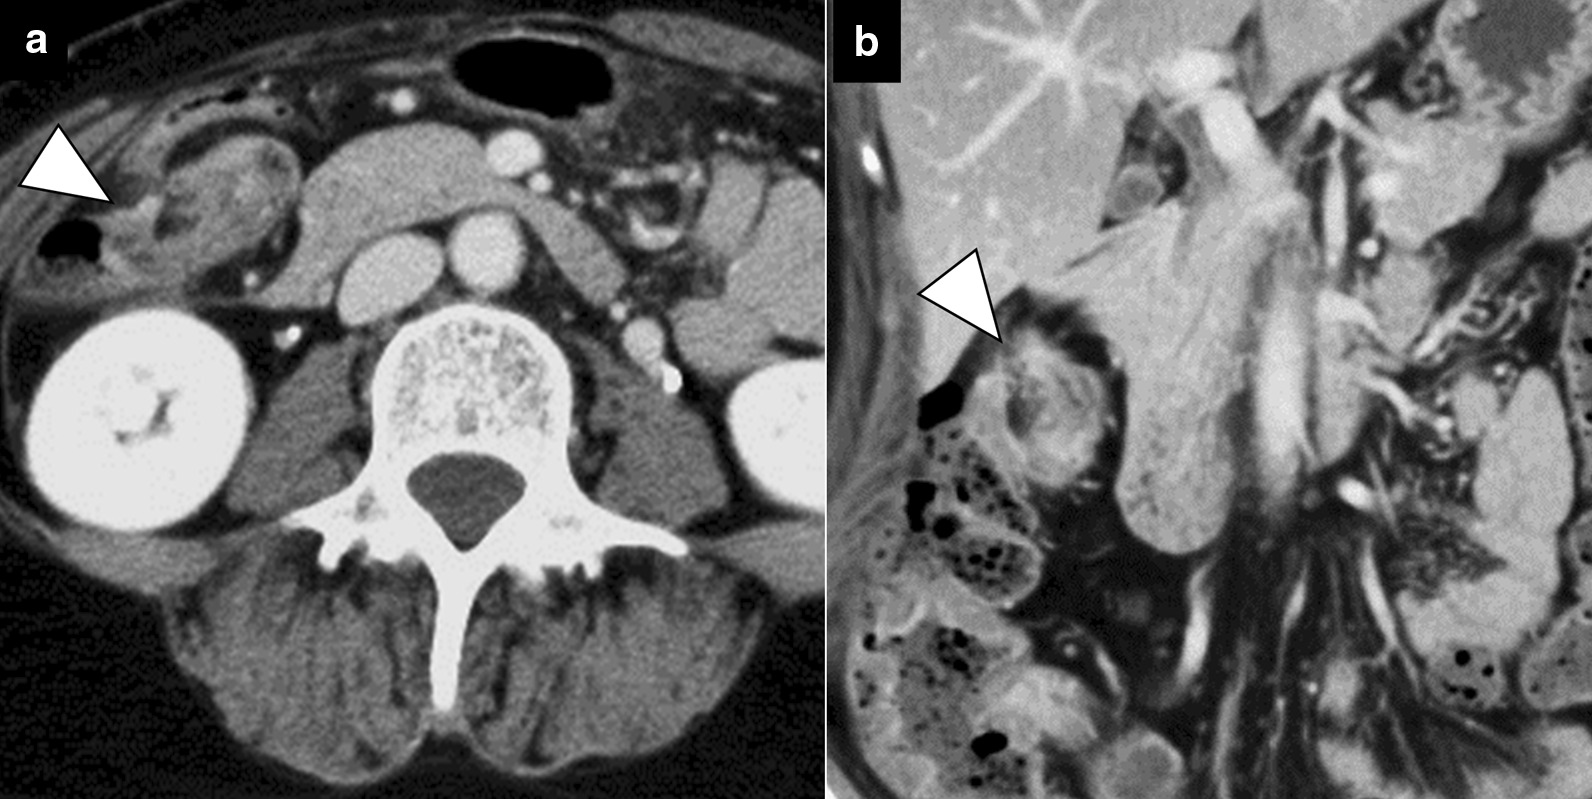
Contrast-enhanced computed tomography showing intestinal torsion at the hepatic flexure (arrowheads) (a; axial image, b; coronal image)
